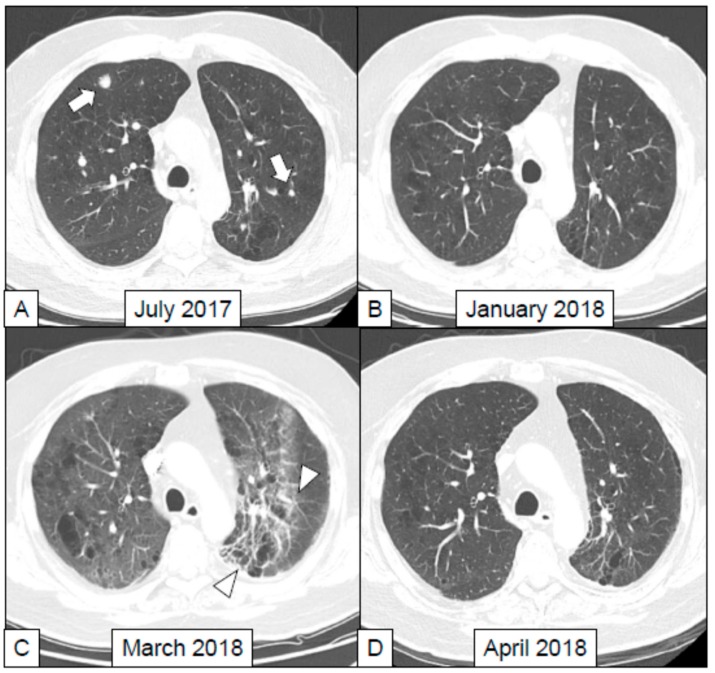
A 64 years old male, a former heavy smoker, was diagnosed with adenocarcinoma of the left lung with multiple ispilateral and controlateral lung metastases and a bone metastasis in the femur. This patient was treated with the anti-PD-1 nivolumab (3 mg/kg q2w) administered as a second line treatment for the metastatic disease. This treatment was given at the Department of Medical Oncology and Hematology, Regional Hospital of Aosta, Italy. (A) The lung CT scan performed in July 2017 before the beginning of immunotherapy shows the presence of pulmonary metastases in both lungs. (B) Those two metastases were not evident anymore in the CT scan performed 6 months later. The response was classified as partial (iPR) according to the iRECIST 1.1 criteria (42). (C) One month after the last CT scan, the patient developed sudden fatigue and dyspnea, with peripheral oxygen saturation equaling to 80%, with no fever and normal circulating levels of markers of systemic inflammation. A lung CT was performed in March 2018 (8 months after the beginning of immunotherapy) showing diffuse interstitial thickening associated with ground-glass pattern that was more evident in the posterior lobar regions. This aspect was suspicious for ir-pneumonitis. (D) The patient was treated with high dose methilprednisolone (1 mg/kg) with improvements in respiratory symptoms, and resolution of the lung pathological findings, as shown by the follow-up CT scan performed in April 2018.
